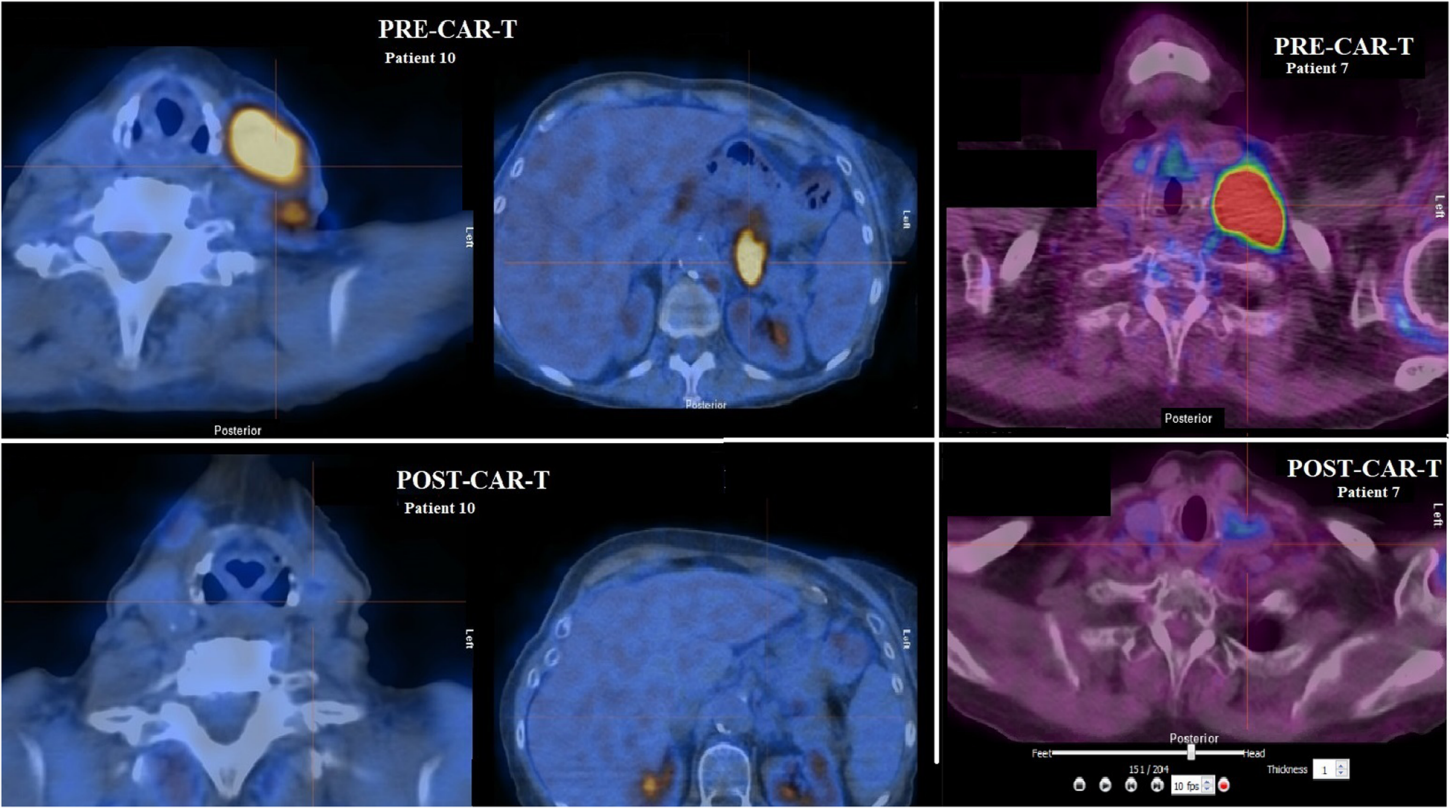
PET-CT images of patients treated with axicabtagene ciloleucel. Pre-CAR-T (above) ad post-CAR-T (below) images show improvement in left cervical and left paraaortic lymphadenopathy in patient 10 and improvement in left cervical lymphadenopathy in patient 7
